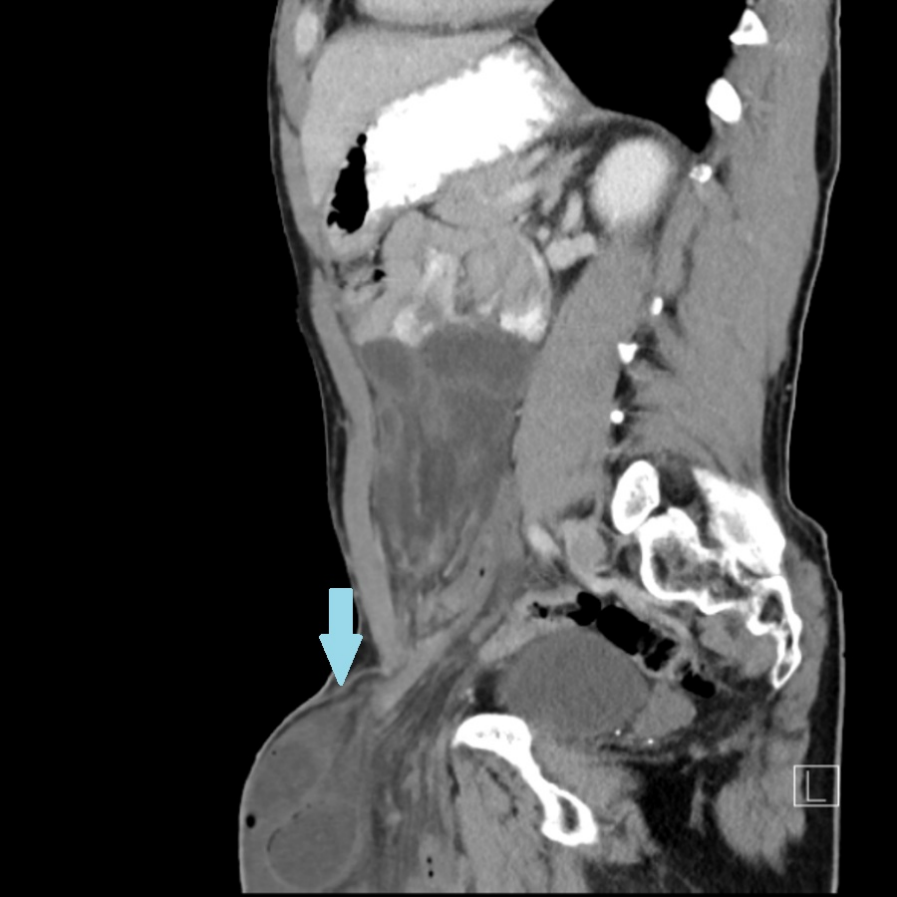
Abdominal computed tomography, sagittal plane Left inguinal hernia (arrow) contains dilated small bowel loops. Infiltration of fat and engorgement of herniated vessels and fluid are also noted.
